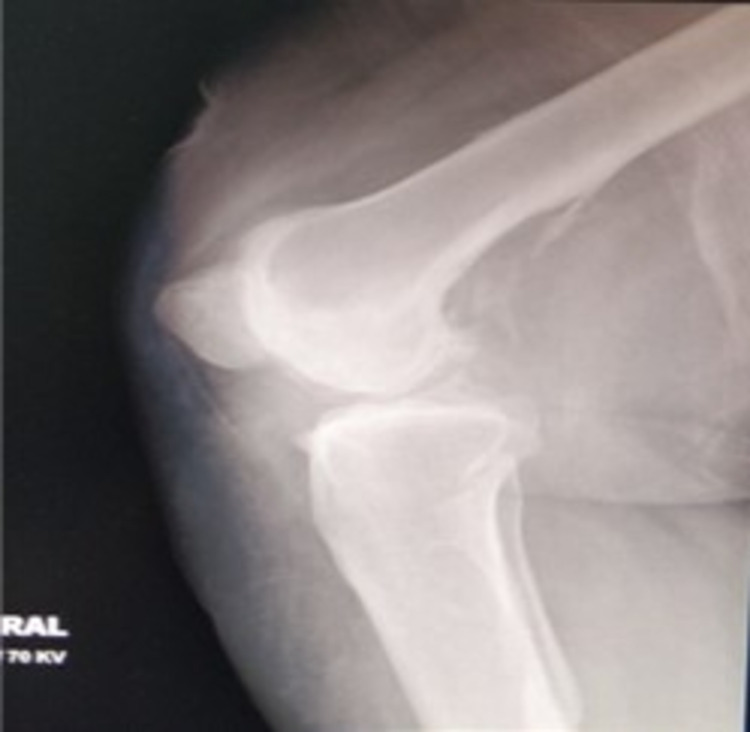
X-ray of the knee: lateral view.
